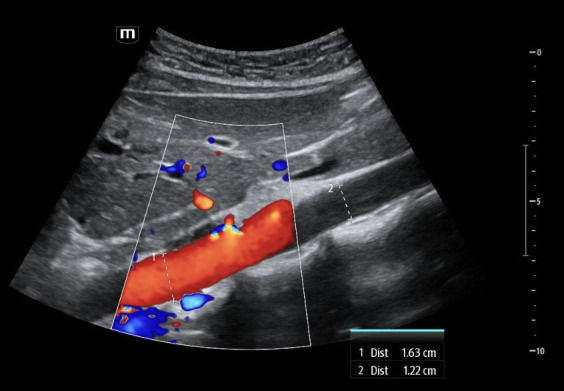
Point of care ultrasound (POCUS) images with color Doppler of a 27-year-old female standardized patient showing a sagittal view of the abdominal aorta. Color flow clarifies which of two neighboring linear hyperechoic structures is the true wall of the abdominal aorta.
